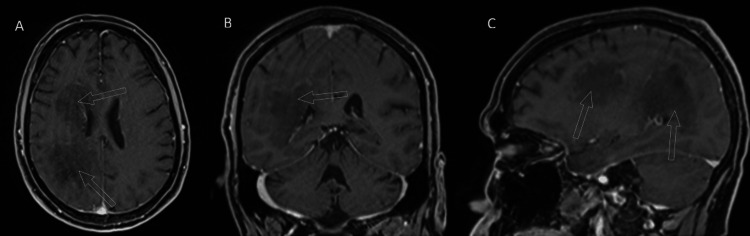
First MRI following treatment Repeat imaging following corticosteroid therapy showed near-complete resolution of the peripherally enhancing lesion of the right parietal lobe, with the areas of interest marked by the unfilled arrow. The axial (A), coronal (B), and sagittal (C) planes are displayed. There is still evidence of persistent mass effect as suggested by effacement of the right lateral ventricle.
